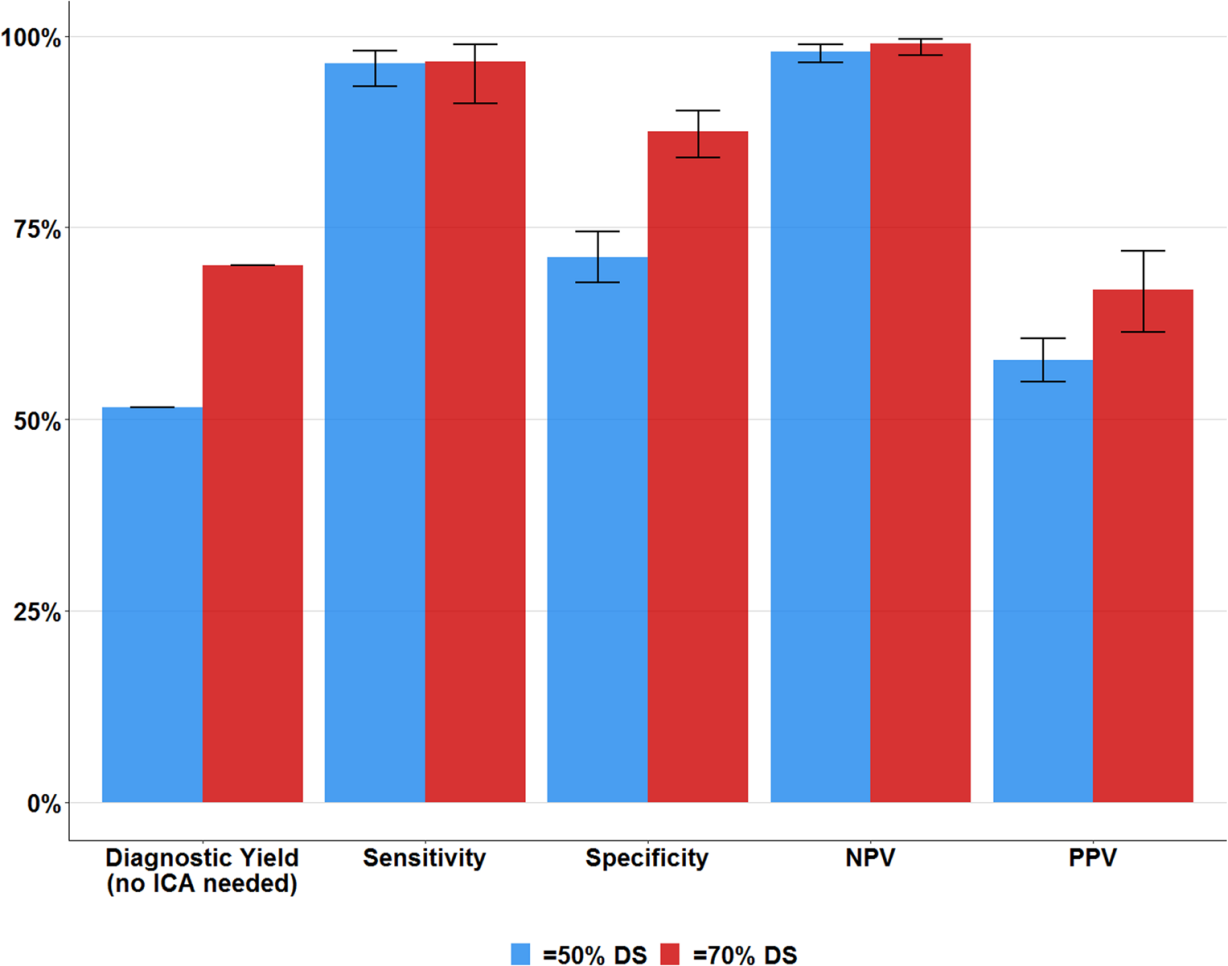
Diagnostic yield and accuracy of CTA to detect proximal coronary stenosis, using a threshold of ≥ 50% and 70% diameter stenosis. Abbreviations: ICA, invasive coronary angiography; CTA, computed tomography angiography; NPV, negative predictive value; PPV, positive predictive value; TAVI, transcatheter aortic valve implantation
